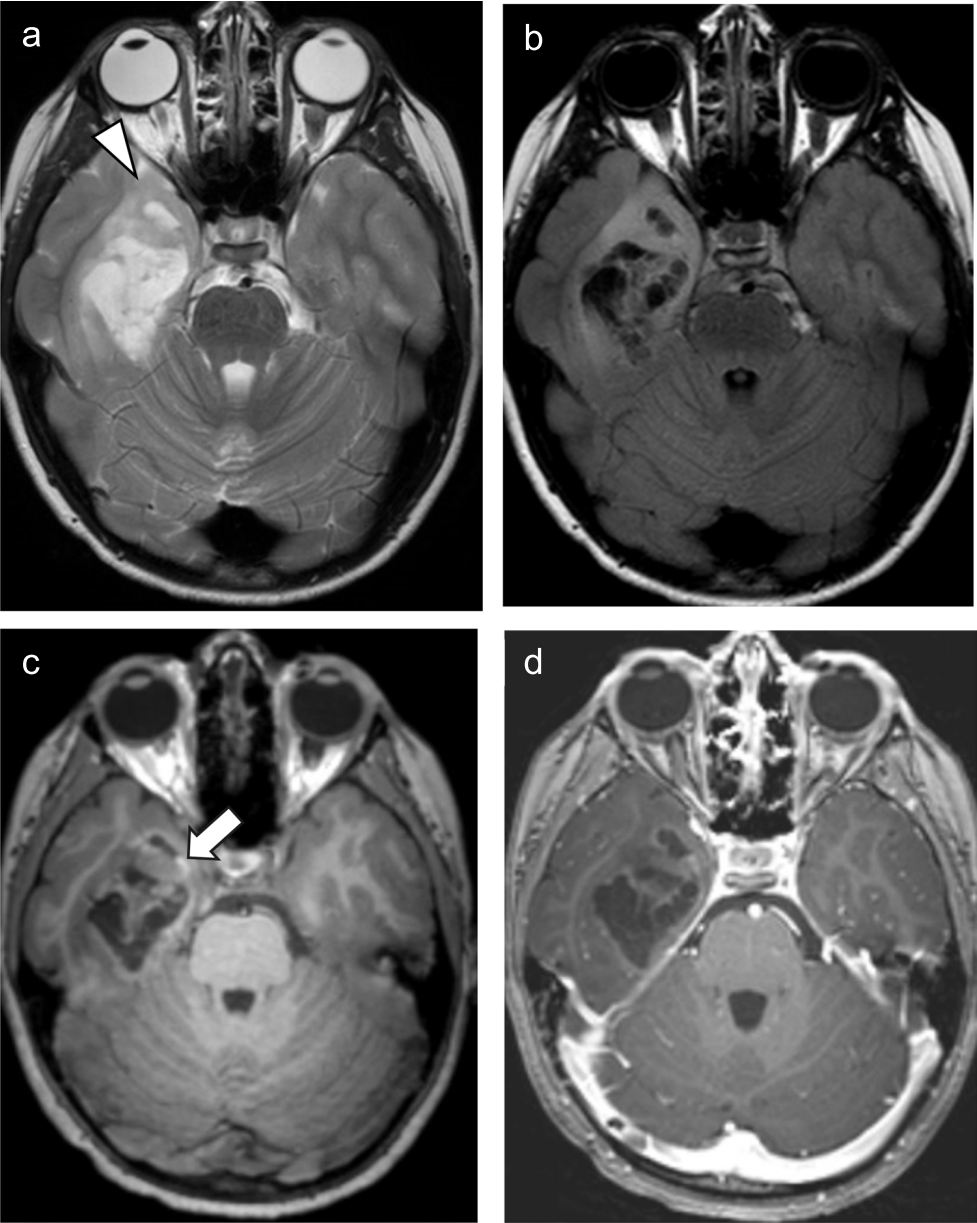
Angiocentric glioma *MYB* and *MYBL1*-altered in an 8-year-old male with a 3-year history of epilepsy. Axial T2-weighted (a), T2 FLAIR (b), T1-weighted (c), and T1-weighted contrast-enhanced (d) MR images show a non-enhancing, cortically-based tumor with subtly indistinct margins anteromedially (*arrowhead*). The T2-FLAIR mismatch sign is present and there is a thin intrinsic T1-hyperintense rim (*arrow*)
